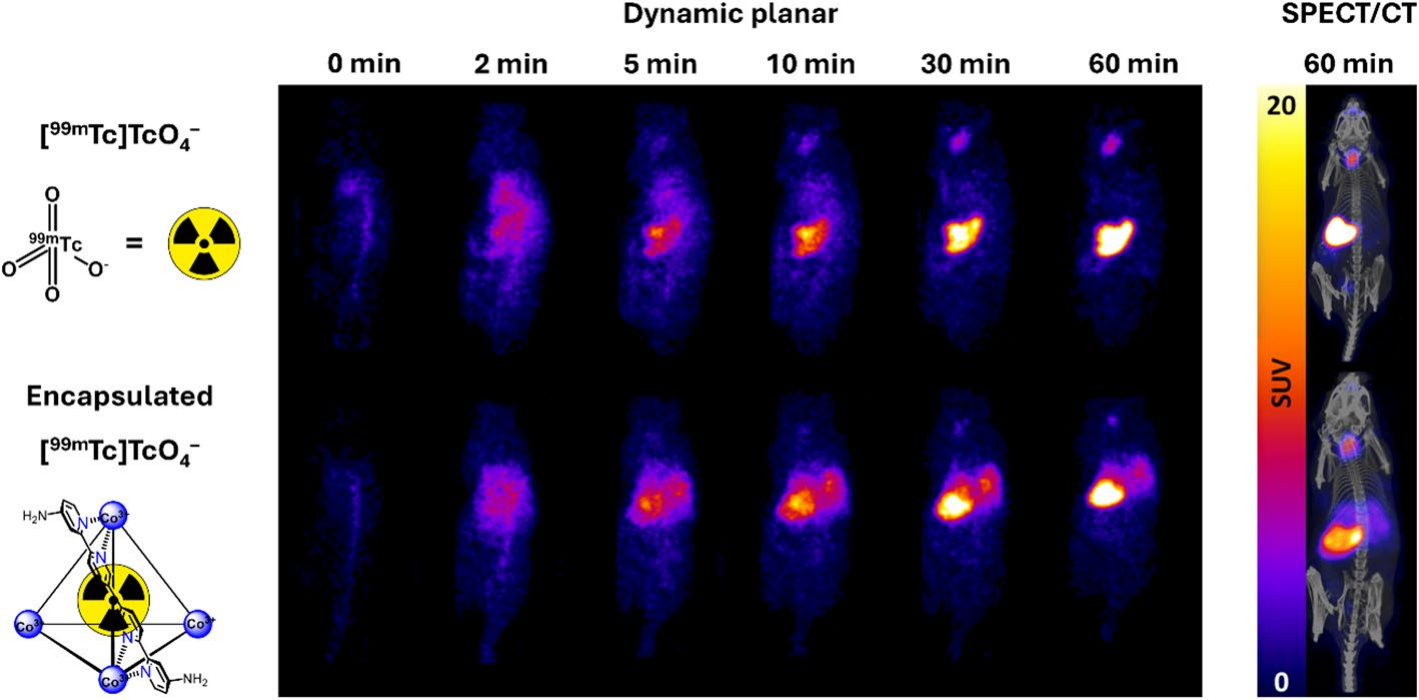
Representative planar scintigraphy and SPECT/CT images of [^99m^Tc]TcO_4_^−^ and [^99m^Tc]TcO_4_^−^BAB cage at selected timepoints
